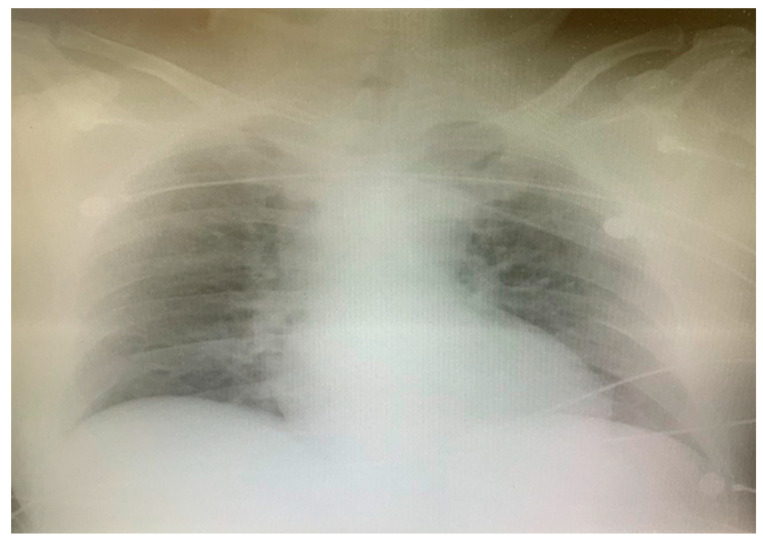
The admission chest X-ray.
